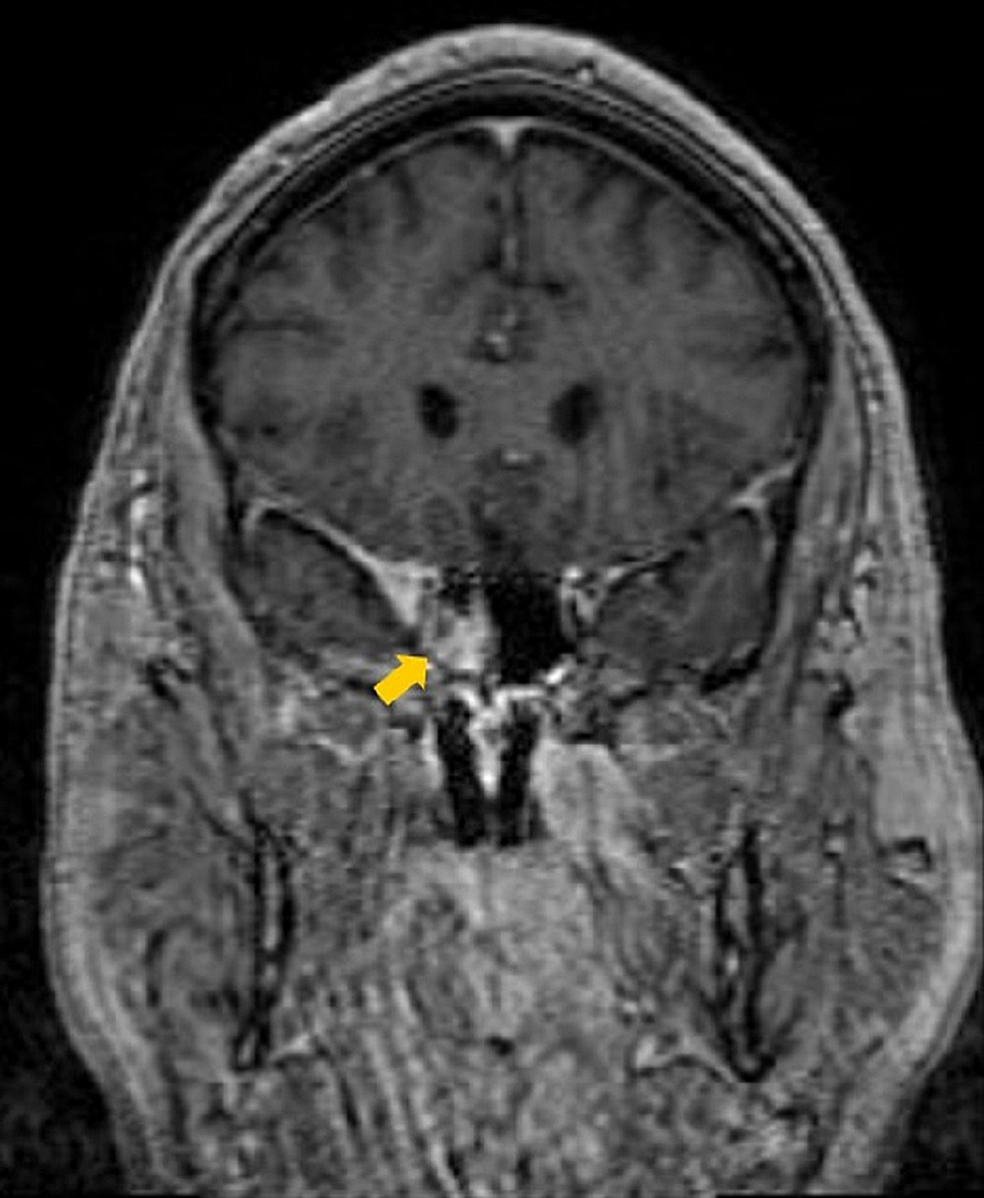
Initial coronal contrast-enhanced T1-weighted MRI The image is demonstrating enhancement of the right cavernous sinus extending into the right orbital apex (yellow arrow).
